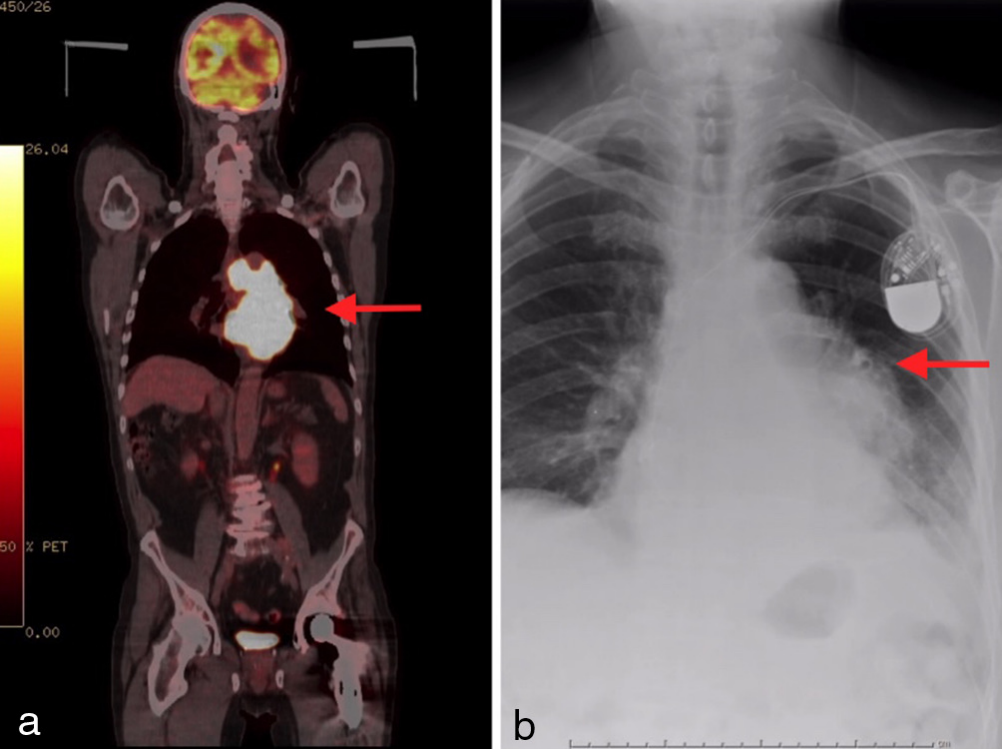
(a) Positron emission tomography showing an infiltrative mediastinal mass with very high intensity fludeoxyglucose uptake. (b) Chest X-ray showing a lobulated mass (lymphoma) at the left hilum associated with small left pleural effusion.
